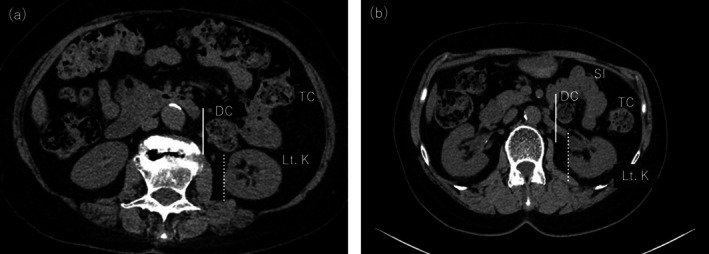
Axial CT images at the level of the left kidney. (a) Case 1, (b) Case 2: The descending colon is medially displaced. The right edge of the descending colon (solid line) is located more medially than the right edge of the left kidney (dotted line). DC, descending colon; Lt.K, left kidney; SI, small intestine; TC, transverse colon.
